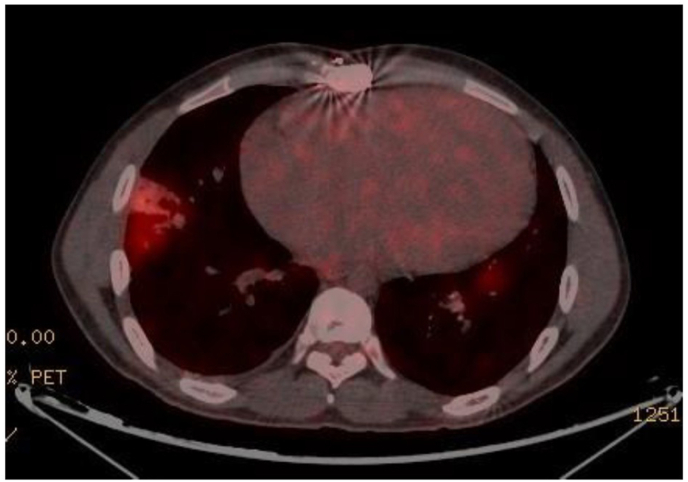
PET-CT showing a right pulmonary embolism.
